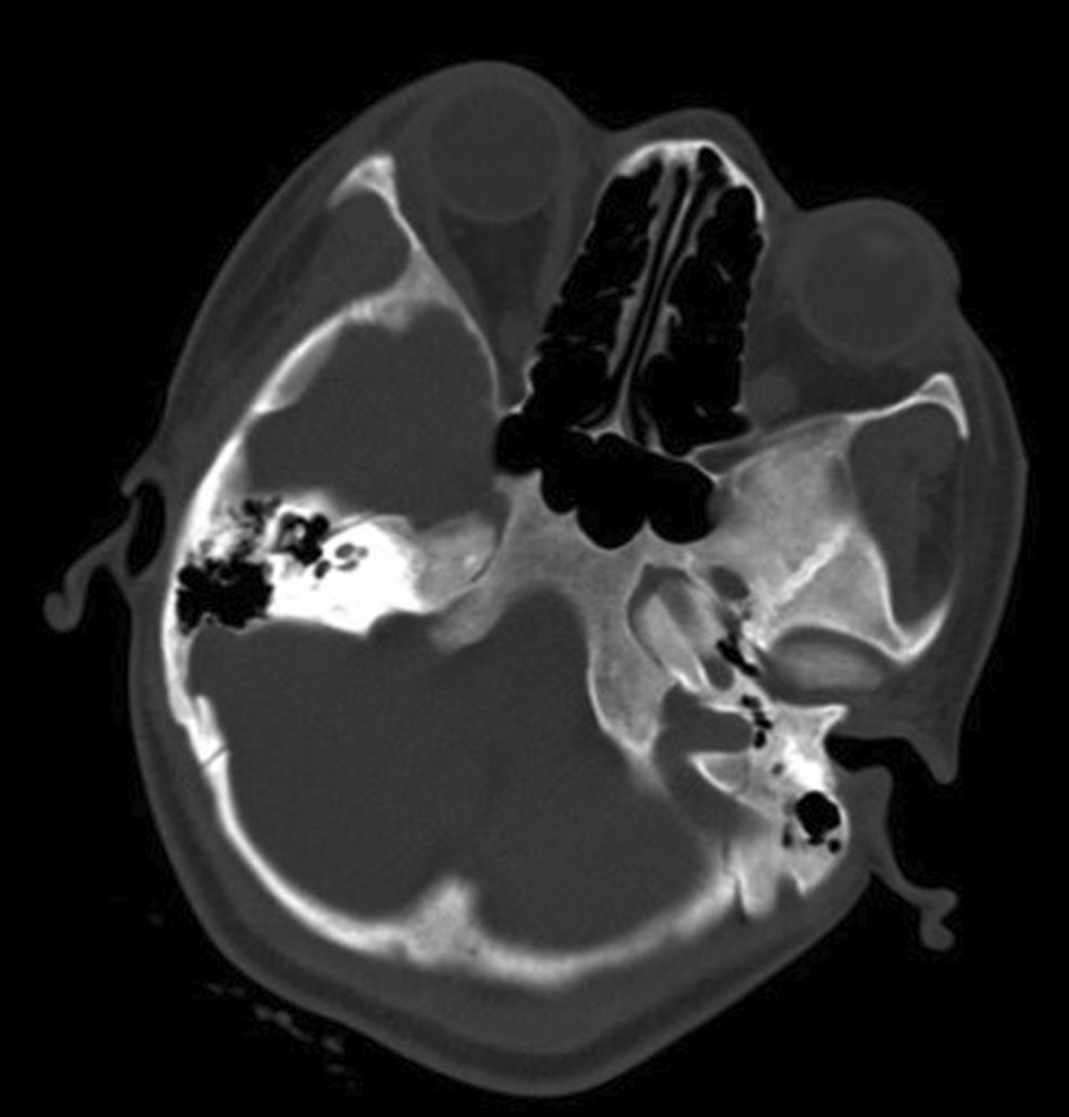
Oculocerebral CT scan with bone window: total disappearance of exophthalmos
